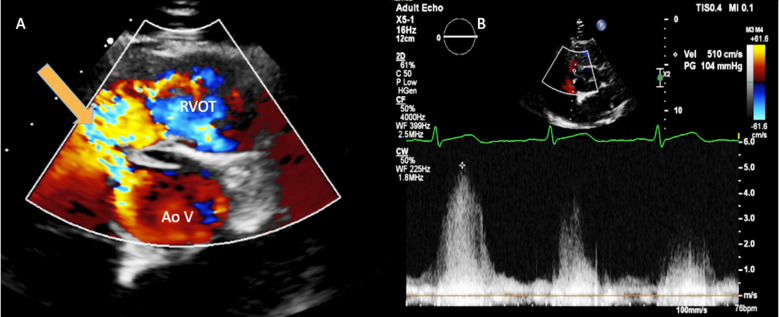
Panel A: Parasternal short axis view with colour Doppler showing the left to right jet through the VSD (red arrow). Panel B: Continuous wave Doppler trace on parasternal short axis view through the jet indicating high velocity left to right flow at 5.0 m/s consistent with a restrictive VSD. VSD, ventricular septal defect.
